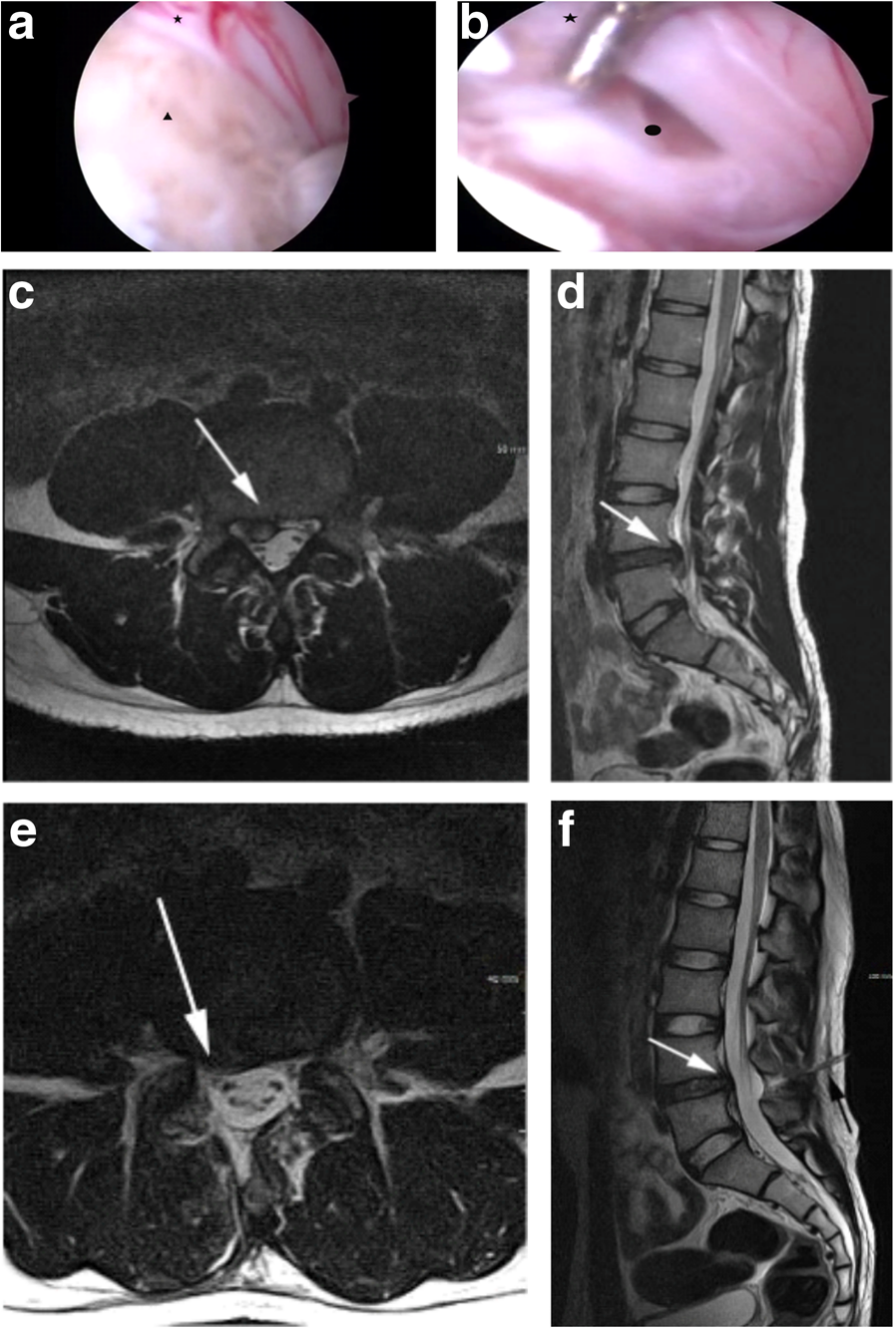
Large disc herniation at the L4–5 level. Intraoperative imaging during unilateral biportal endoscopic (UBE) discectomy of a 28-year-old man presenting with severe back and right leg radiating pain. The herniated disc triangle compressing the thecal sac and traversing nerve root star (a), thecal sac, traversing nerve root star, and the posterior ligament circle were freely movable after disc fragment removal (b). Magnetic resonance (MR) axial image shows paracentral disc herniation and compression in the thecal sac and L5 traversing nerve root (white arrow) (c). Sagittal MR image shows down migrated disc compressing the thecal sac (white arrow) (d). After UBE discectomy, postoperative axial MR image shows decompressed thecal sac and traversing L5 nerve root (white arrow) (e). Postoperative sagittal MR imaging shows removed herniated disc (white arrow) and minimally invasive instrumental pathway for discectomy (black arrow) (f)
